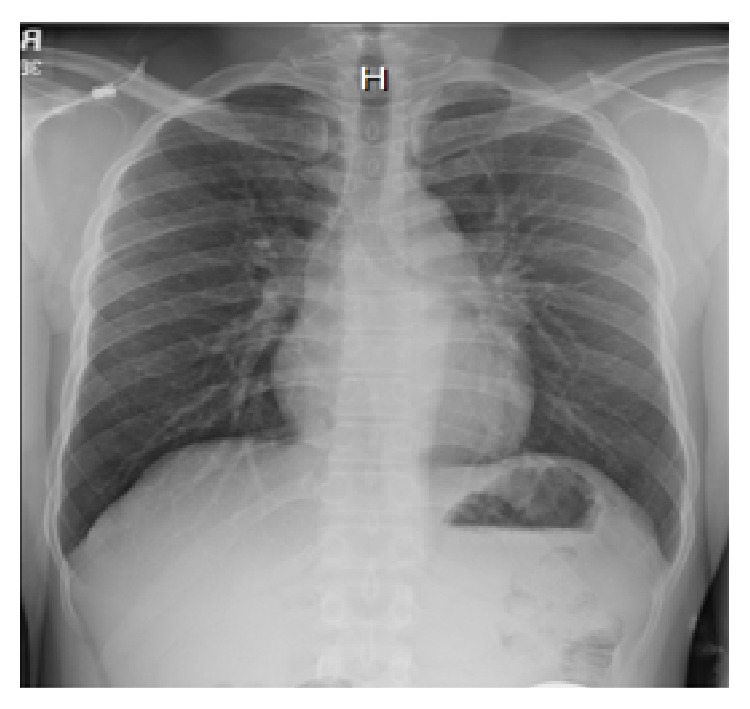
Chest X-ray (PA view) with left hilar fullness but no pulmonary lesions.
